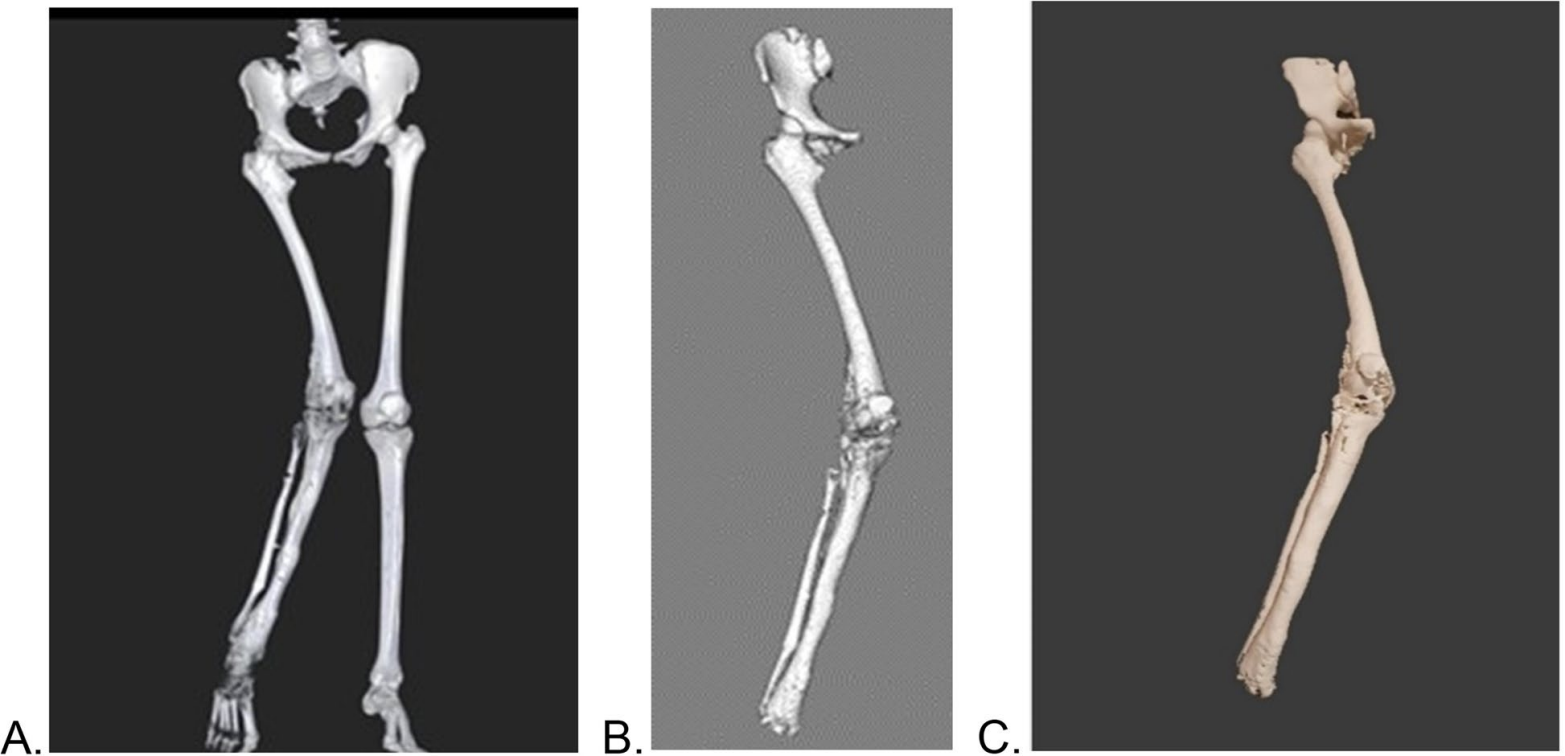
A) Pre-surgery CT scan of patient, B) Anatomage MD Studio anatomical models of the defect area, and C) 3D printed prototype model of the anatomical defect
